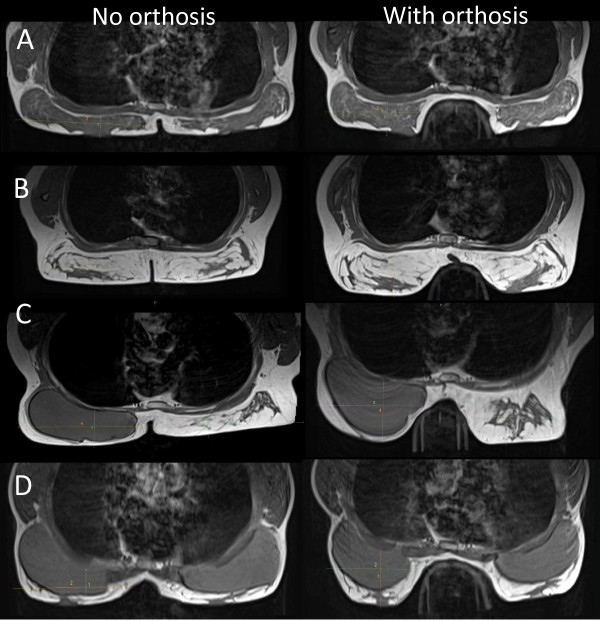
Assessment of breast displacement. MRI transverse view of natural breast C-cup (A); lactating breast EE-cup (B); unilateral reconstruction C-cup (C); primary augmentation DD-cup (D); all without (left panel) and with orthosis (right panel).
